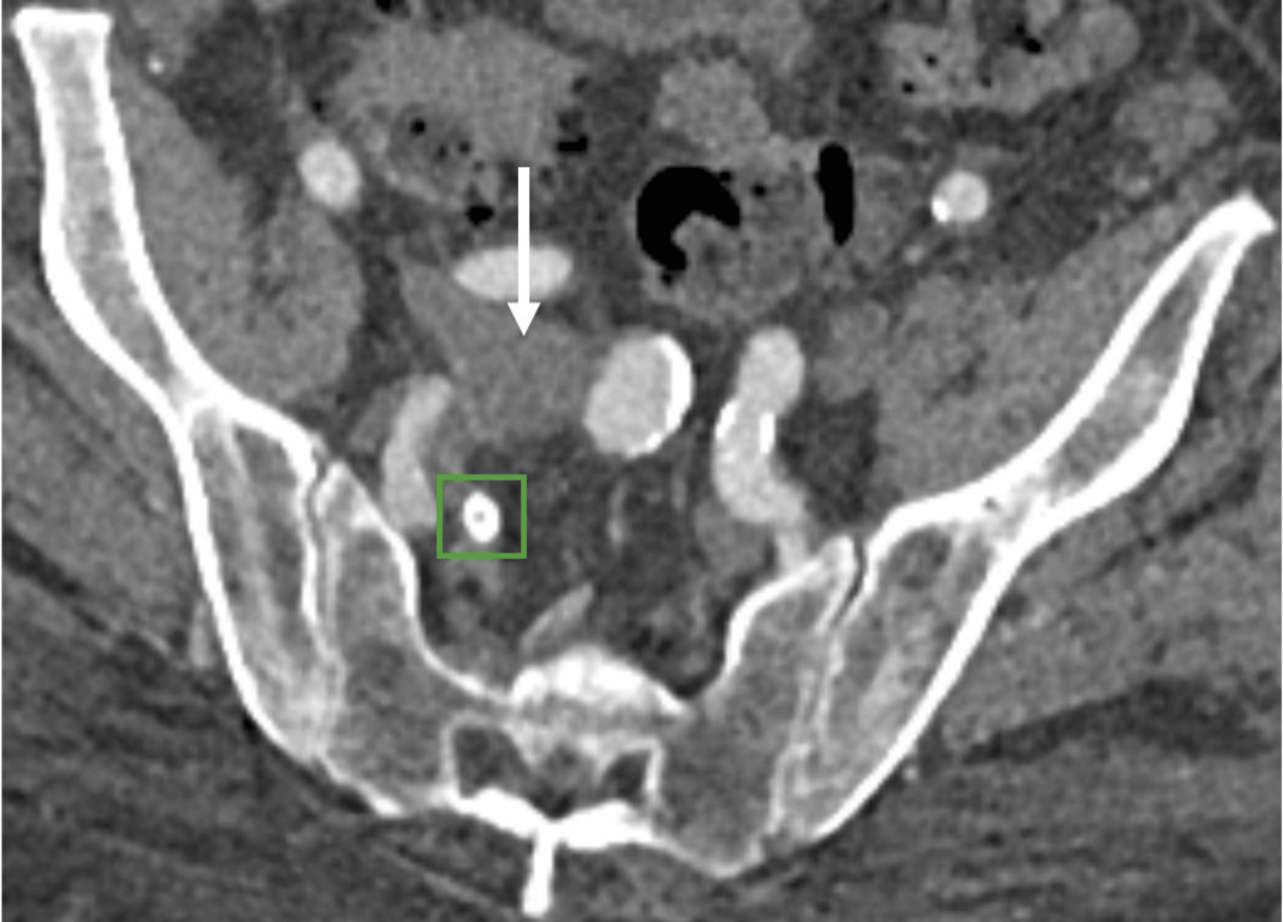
Post-embolization follow-up CTA abdomen pelvis demonstrates decreased caliber of the right common iliac vein (CIV; white arrow), with the plug seen in the previous fistulous connection (green box). In this arterial phase image, no early filling of the CIV is seen in comparison to pre-embolization. CTA: Computed tomography angiography.
